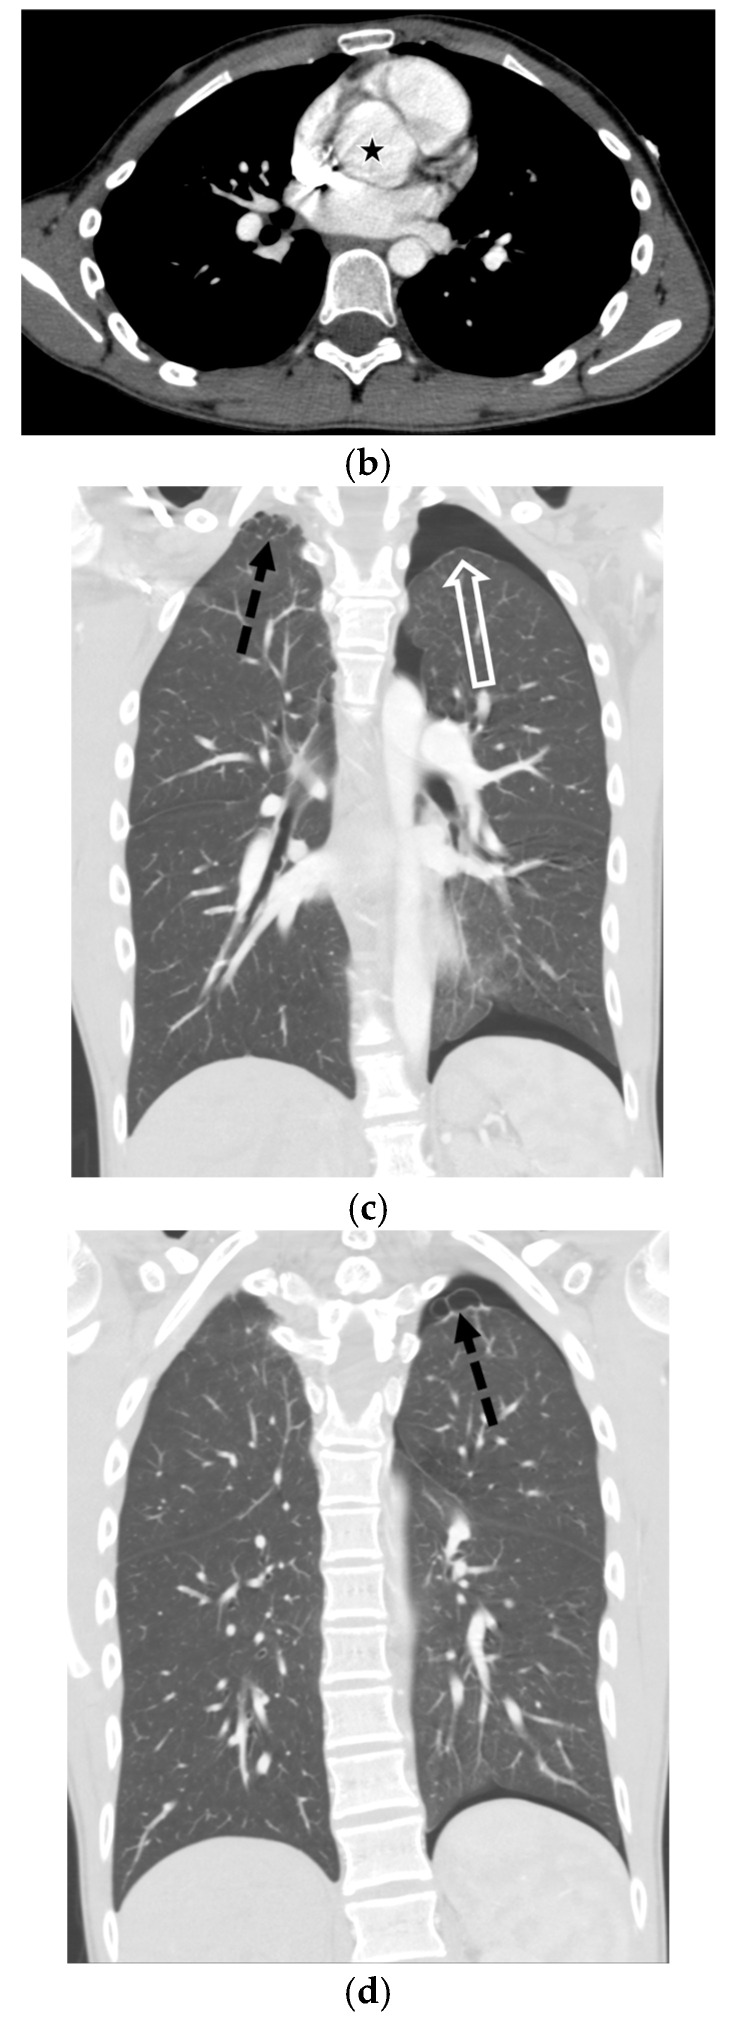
16-year-old male with Marfan’s syndrome presents with left-sided chest pain. Frontal chest radiograph (a) demonstrates moderate left pneumothorax (open black arrow) along with increased craniocaudal-to-transverse ratio of the thorax. Axial contrast-enhanced CT image in soft tissue window (b) shows dilated aortic root (black star). Coronal CT images in lung window (c,d) reveal biapical blebs (dashed black arrow) and moderate left pneumothorax (open white arrow).
